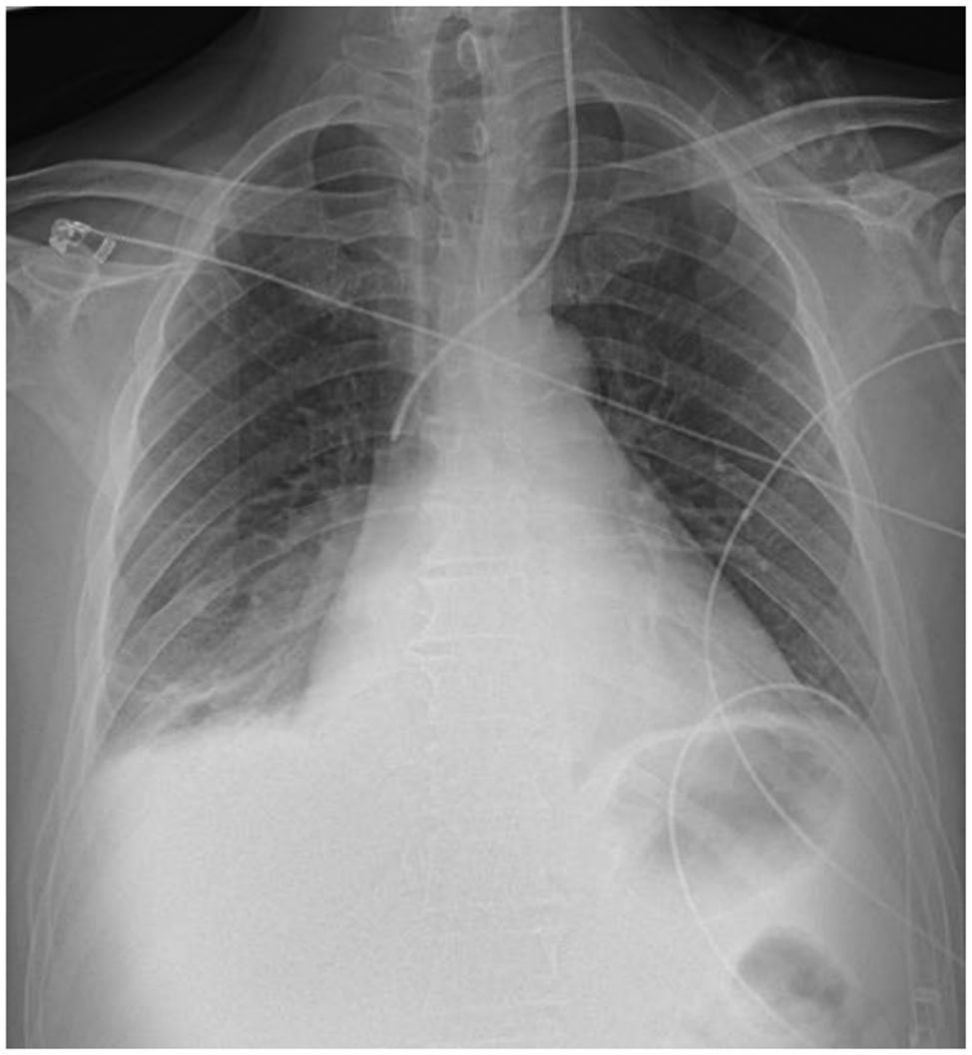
Plain chest X-ray findings: Hypolucency is noted in the right lower lung field
